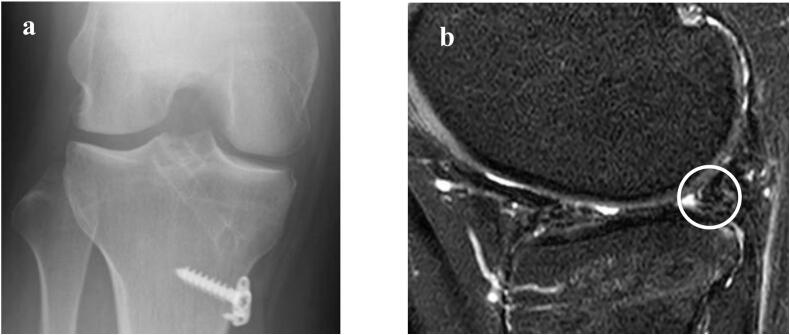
Postoperative imaging at 24 months. a Rosenberg view X-ray of the lateral compartment shows lateral joint space widening to 3.7 mm. b Sagittal magnetic resonance imaging (MRI) demonstrates the autograft in the posterior part of the lateral meniscus (white circle).
